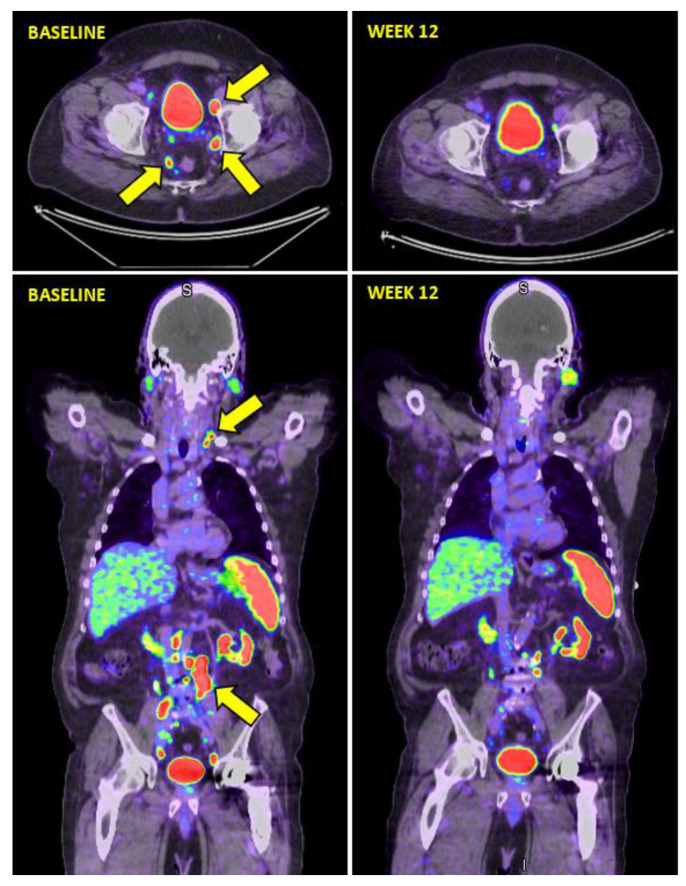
Example of week-12 ^68^Ga-PSMA-11 PET/CT response assessment following ^177^Lu-PSMA I&T for mCRPC. Baseline ^68^Ga-PSMA-PET/CT (left panels) nodal metastases involving left cervical chain and multiple abdominopelvic nodes (yellow arrows). Week-12 ^68^Ga-PSMA-PET/CT response assessment (right panels) showing a near complete response with resolution of the majority of nodal disease above and below the diaphragm. Consistent with an excellent partial remission.
